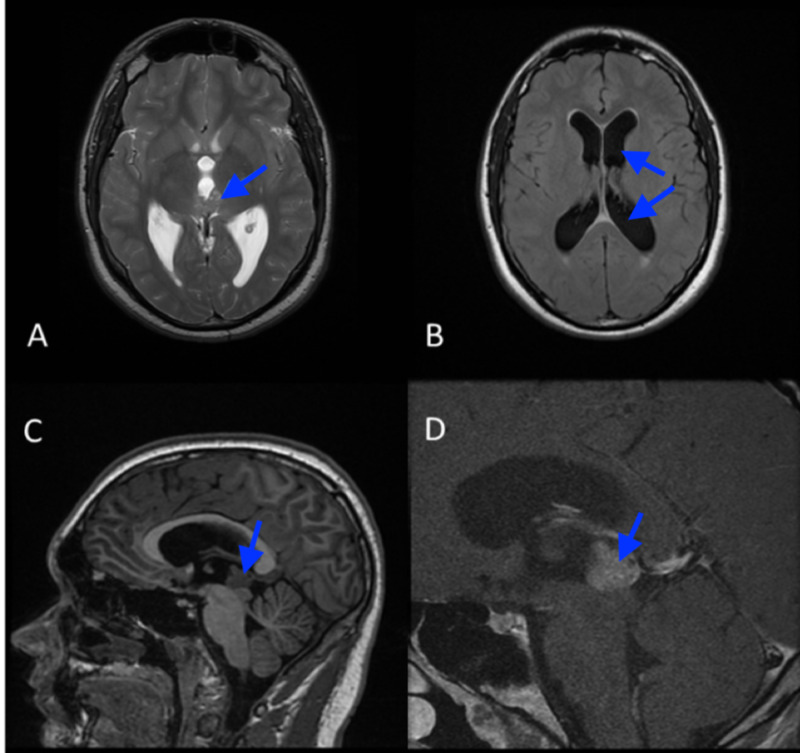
Pre-treatment MRI of the brain. Axial T2-weighted MR image (A) of the brain at the level of the basal ganglia shows a predominantly T2 hypointense mass involving the pineal region (arrow), which causes a mass effect on the cerebral aqueduct and the third ventricle resulting in hydrocephalus, well seen in the axial FLAIR MR image (B). Sagittal T1-weighted MR image (C) shows the lesion is hypointense on T1 and homogeneously enhances on sagittal post-contrast T1-weighted MR image (D). MRI, magnetic resonance imaging; MR, magnetic resonance; FLAIR, fluid-attenuated inversion recovery
